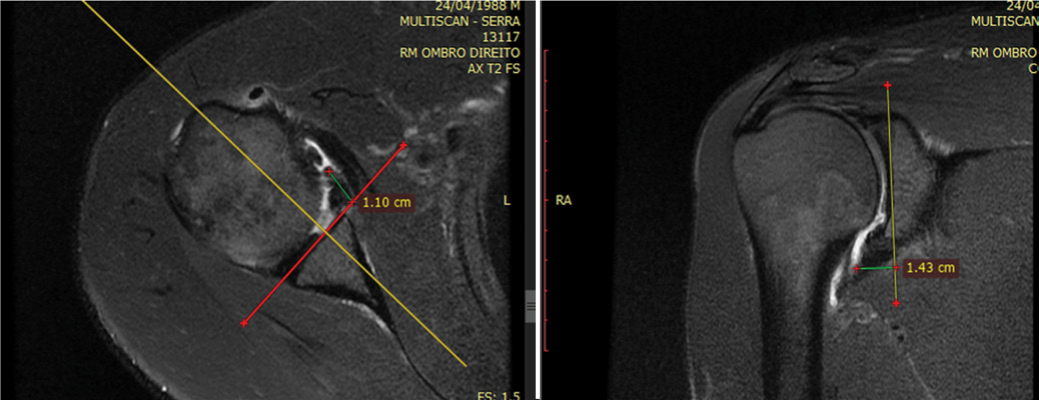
Method for measuring lip height, axial and coronal cuts, using magnetic resonance imaging.
